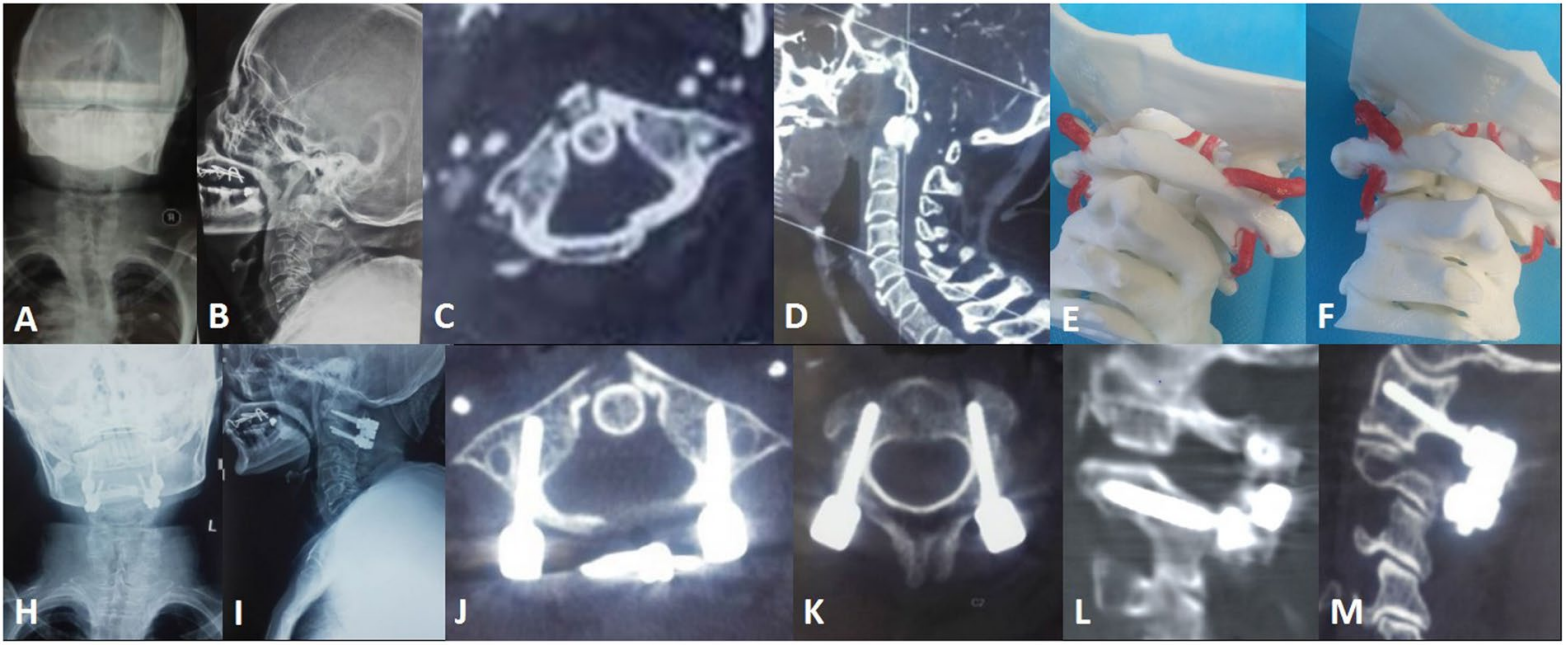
A 55 year-old female with atlantoaxial dislocation underwent posterior reduction and C1 pedicle-C2 pedicle internal fixation assisted by a 3D-printed life-size model. (A–D) Preoperative X-ray photographs showing a Jefferson fracture and odontoid fracture. (E) 3D life-size model showing that the vertebral artery on the left side was closer to the middle line and revealing the best screw trajectory. (I–M) Postoperative photographs showing that the screw position was satisfactory.
